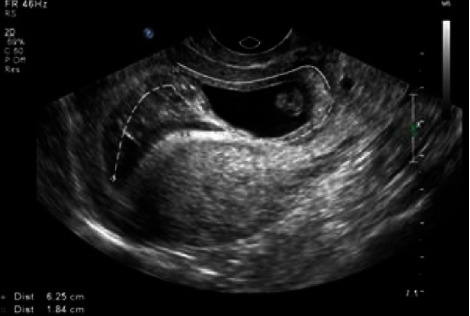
Ultrasound image of the implantation site. A small amount of fluid was observed inside the endometrial cavity, which delineated the border of the hyperechoic ring around the conceptus. The dotted line indicated the endometrial lumen, and the solid line indicated the implantation site.
